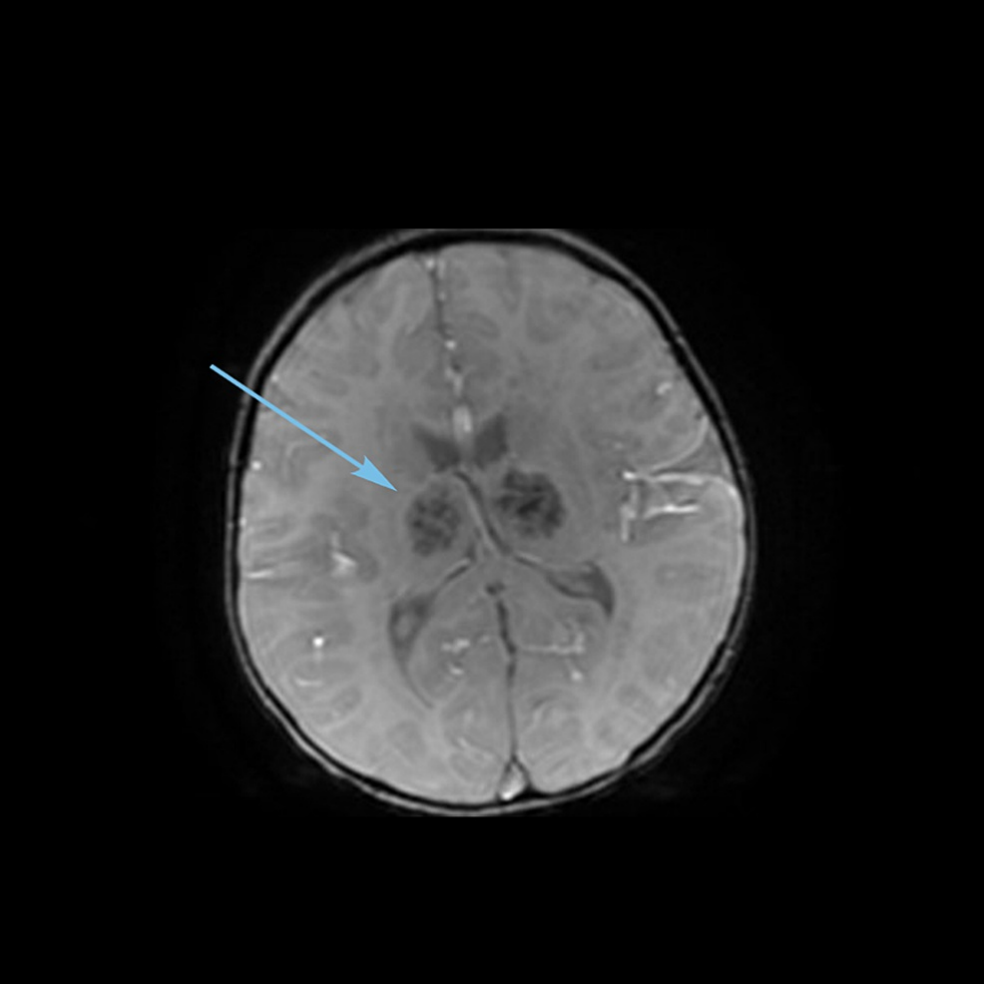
MRI brain axial T2-weighted image showing hyperintensities and edema in bilateral thalami (arrow).
